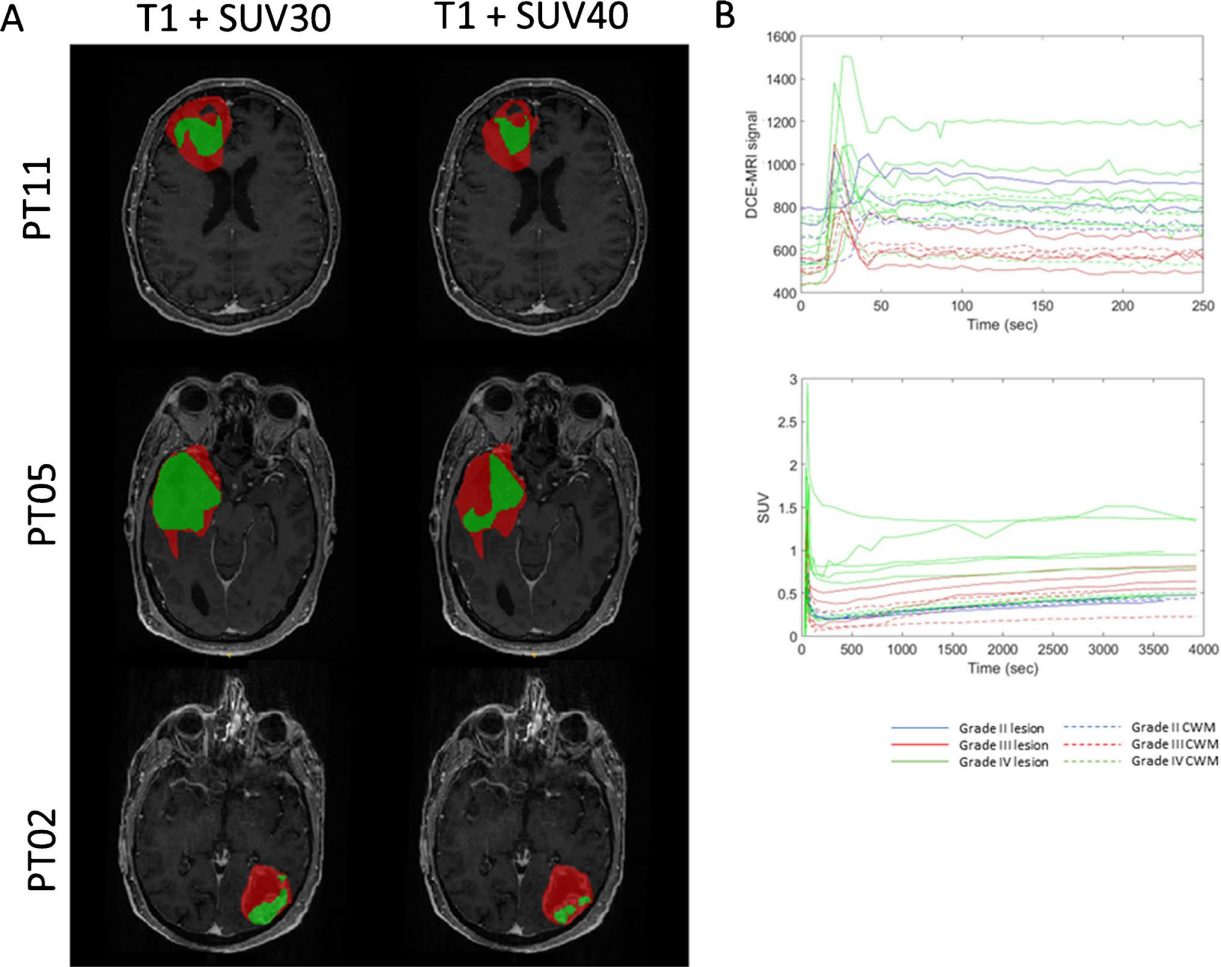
Image congruence. (A) Lesion mask outlined on the post-contrast T1 MRI image (red) and on the SUV_40_ and SUV_30_ (green). (B) Dynamic contrast-enhanced (DCE) MRI and SUV average tissue time activity curves in grade II (blue), grade III (red) and grade IV (green) for lesion or CWM
